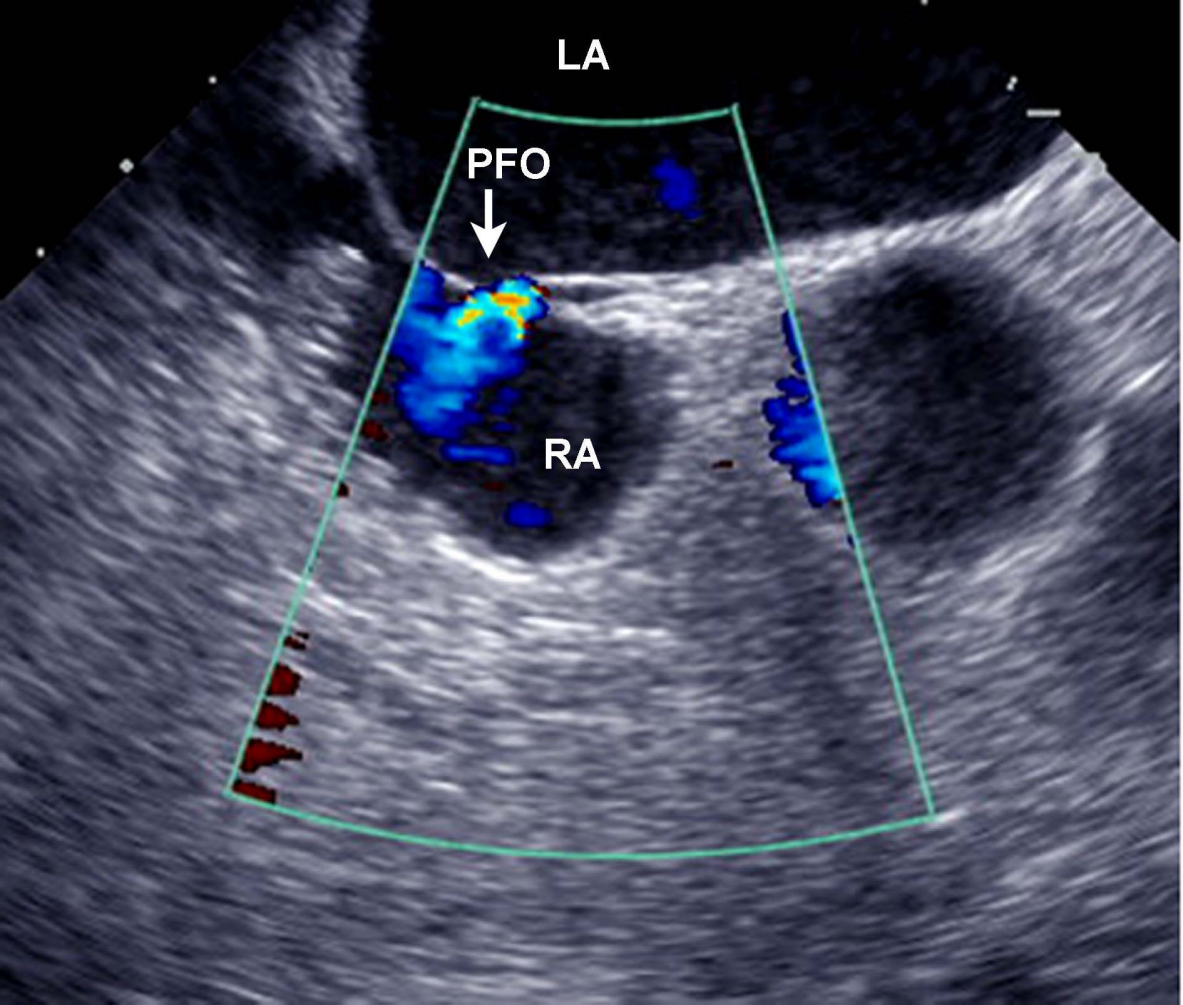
Transesophageal echocardiography. A patent foramen ovale (PFO) with a left-to-right shunt is shown at the level of the interatrial septum. RA and LA indicate right and left atria.
